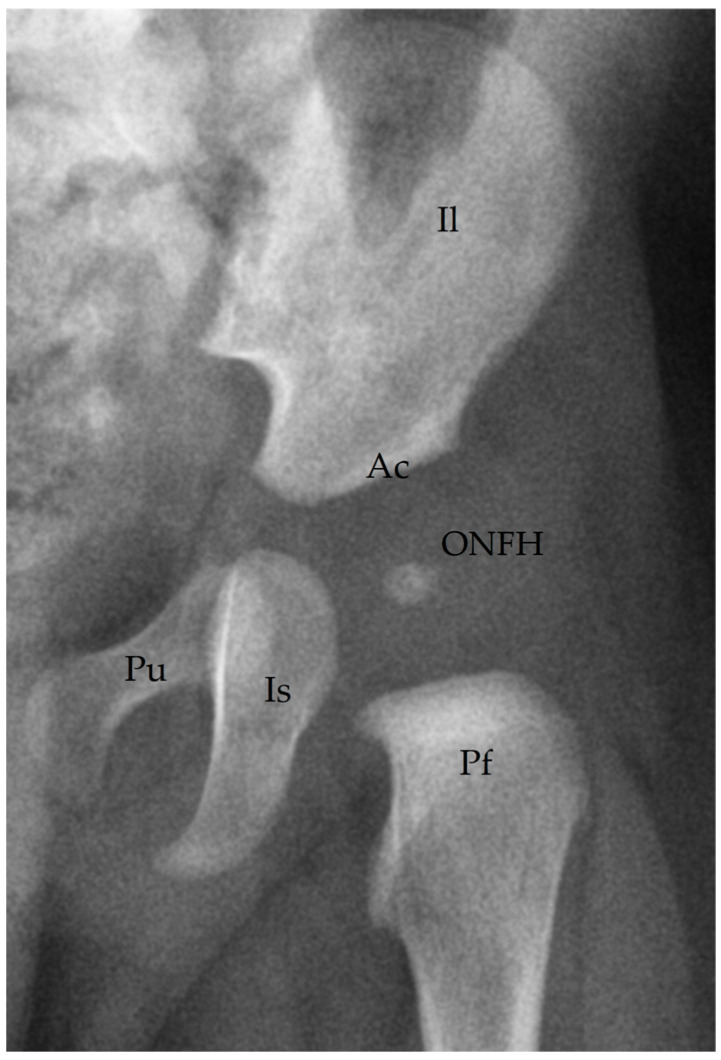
Anteroposterior radiograph of the pediatric pelvis. Il: Ilium; Ac: acetabulum; Pu: Pubis; Is: Ischium; Pf: Proximal Femur; ONFH: Ossification Nucleus of the Femoral Head.
